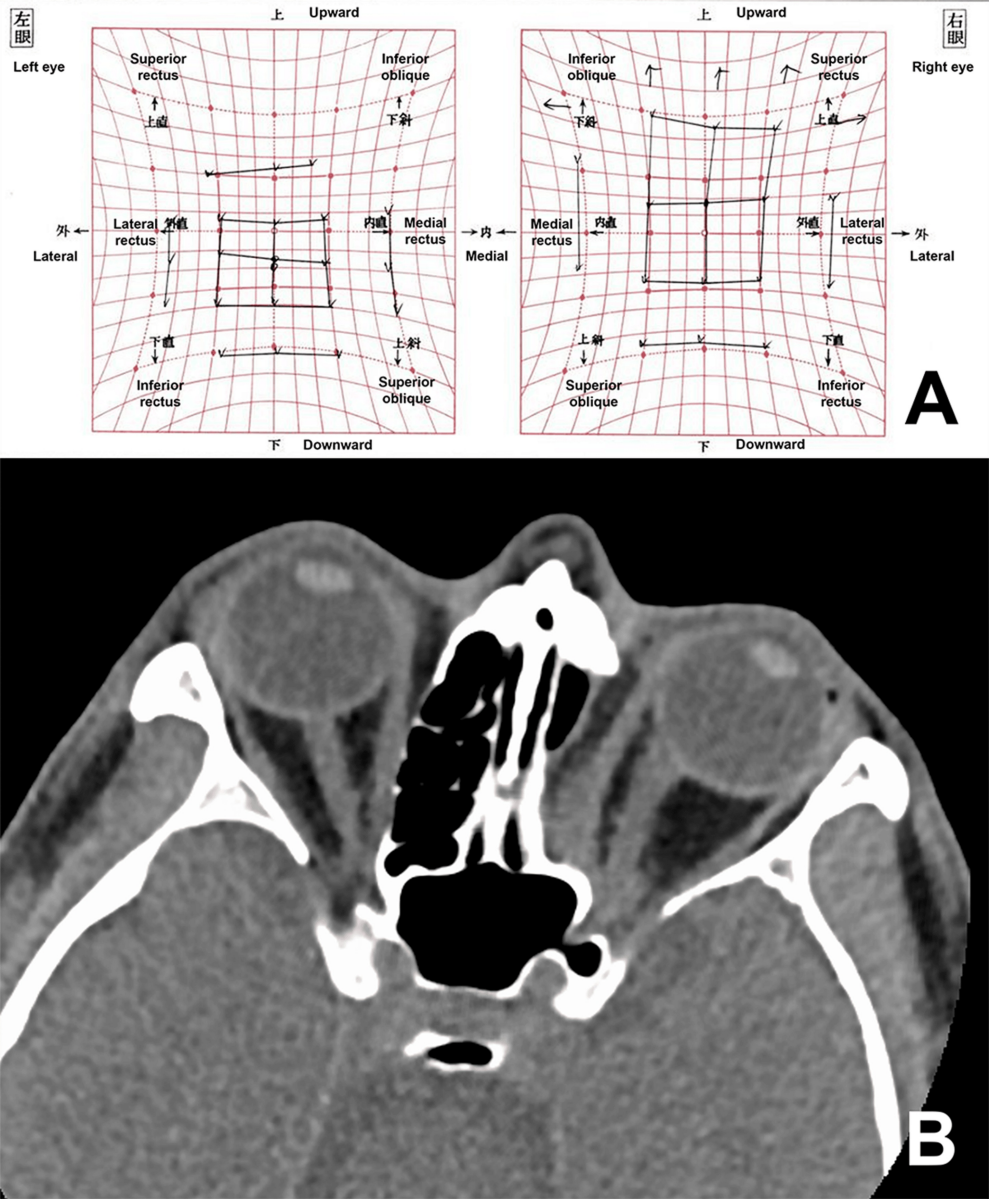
Postoperative findings. A: Hess chart showing no restriction of adduction; B: an axial computed tomographic image taken nine months after surgery showing no recurrence of the tumor and no reconstruction of the medial orbital wall.
